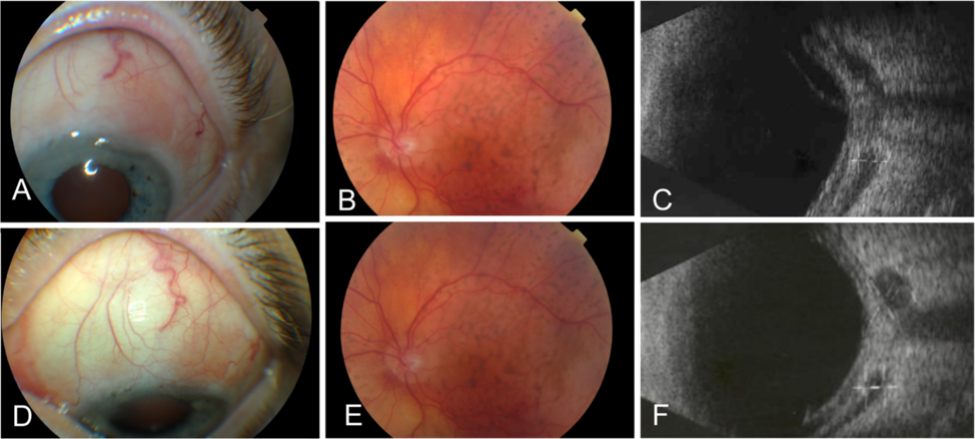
The response to oral chlorambucil at four (A,B,C) and 10 (D,E,F) months after the onset of therapy. The conjunctival lesion is diminished (A,D), with flattening of the choroid together with disappearance of secondary retinal detachment (B,E) and regression of the choroid mass and retrobulbar lesions on ultrasound (C,F).
